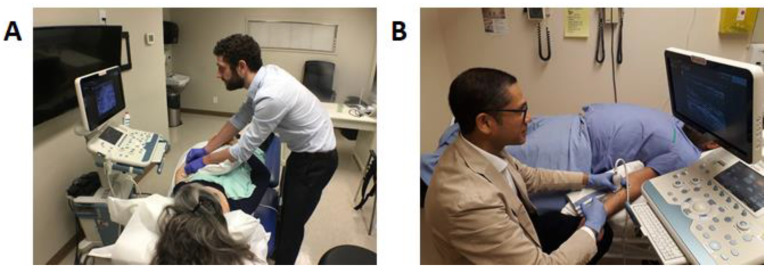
Visual comparison of optimal and sub-optimal ergonomics. (A) Sub-optimal ergonomics: The height of the bed is too low for the injector, requiring forward flexion of the trunk. Because of this positioning and because the ultrasound screen is not at eye level, there is a compensatory extension of the neck, resulting in possible neck strain. In addition, both ultrasound machine and targeted limb are away from the injector, resulting in excessive scapular protraction that can result in shoulder pain. (B) Correct ergonomic positioning: Notice the injector seated with the ultrasound machine on the same side as the targeted limb so that there is a direct line of sight (i.e., ability to see the patient, the ultrasound screen, and the limb). In addition, note the position of the transducer grip used for an in-plane injection to the left upper limb flexor carpi radialis muscle. The patient is comfortable, with the left arm in optimal positioning for injection. Notice the injector’s neutral wrist position of the right hand with no excessive flexion and needle positioning (needle at 25 degrees insertion and approximately 0.5 cm to 1 cm from the transducer). The ultrasound machine is well-positioned within easy reach as to permit the ability for image optimization.
